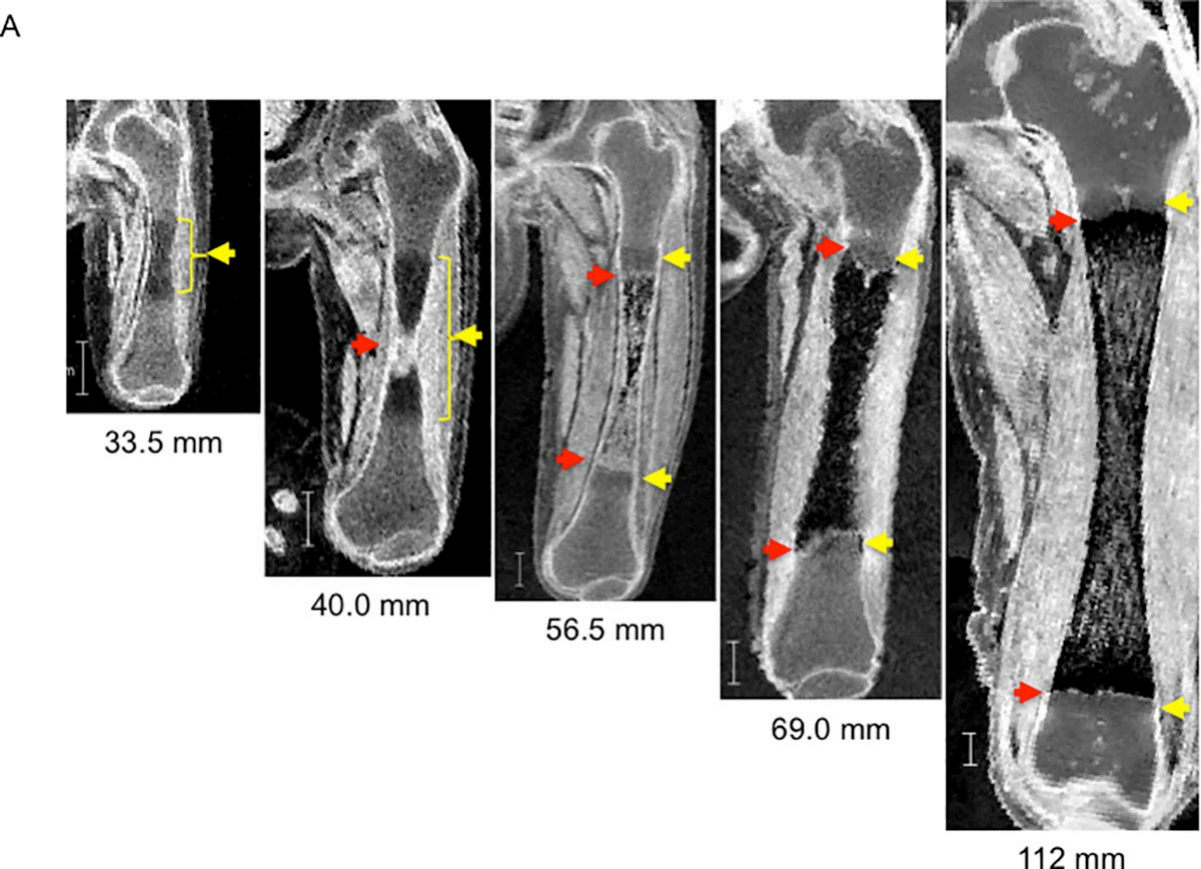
Representative cross-sectional view of the right femur during the early fetal period on 7-T MR image. The crown–rump length (CRL) of each fetus is indicated below the images. Yellow arrow: phase 4; red arrow: phase 5 according to Streeter’s classification. The region between the yellow arrows at the diaphysis corresponds to phase OS. The region outside the yellow arrows corresponds to phases 1–3.
